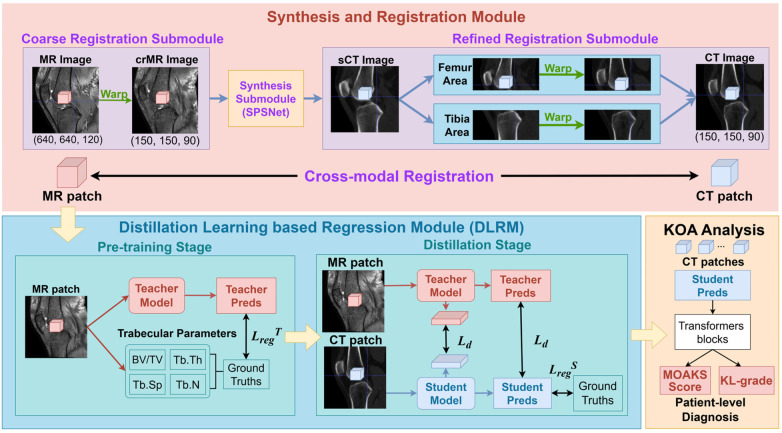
Our CT-SMA framework for cross-modal trabecular parameter regression and subchondral-bone-microstructural-analysis. The synthesis and registration module achieves MR-to-CT alignment through three dedicated submodules. First, a coarse MR-to-CT registration submodule aligns MR images to CT space to generate crMR images. Second, a synthesis submodule based on SPSNet transforms crMR images into sCT while preserving anatomical structures. Third, a refined registration submodule performs component-wise registration from sCT to CT for individual bone regions. With paired MR and CT patches, the distillation-learning-based regression (DLRM) module first pre-trains an MR-based teacher model and then supervises the CT-based student model with knowledge distillation. The regressed parameters are further employed for KOA analysis, including KL-grade-based KOA classification and MOAKS score prediction.
